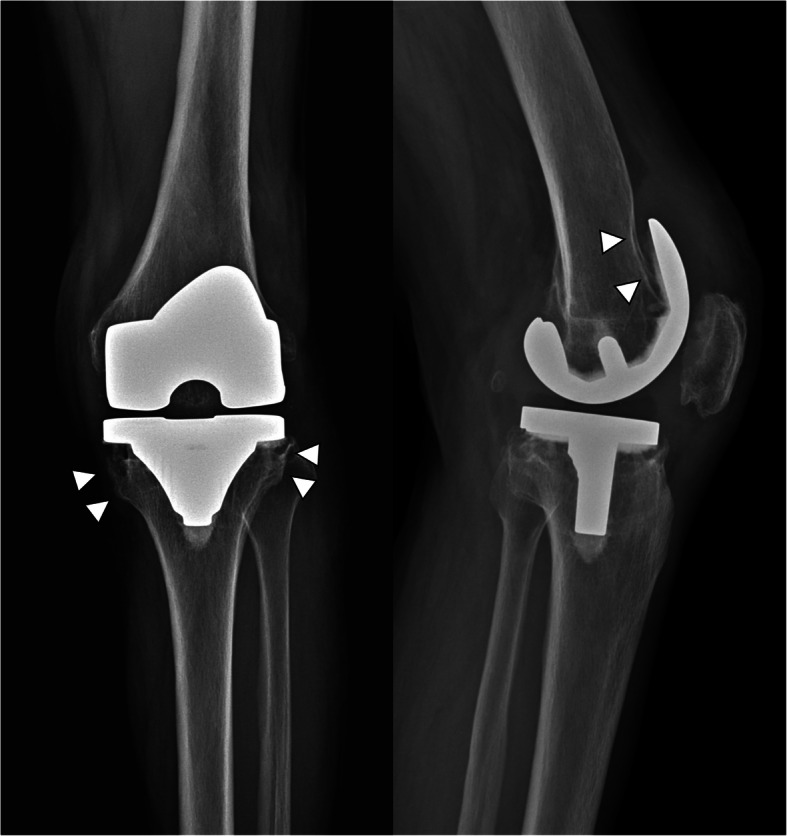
Plain radiography image of anteroposterior and lateral view of the left knee at admission, showing bony resorption of proximal tibia and distal femur (arrow heads)
